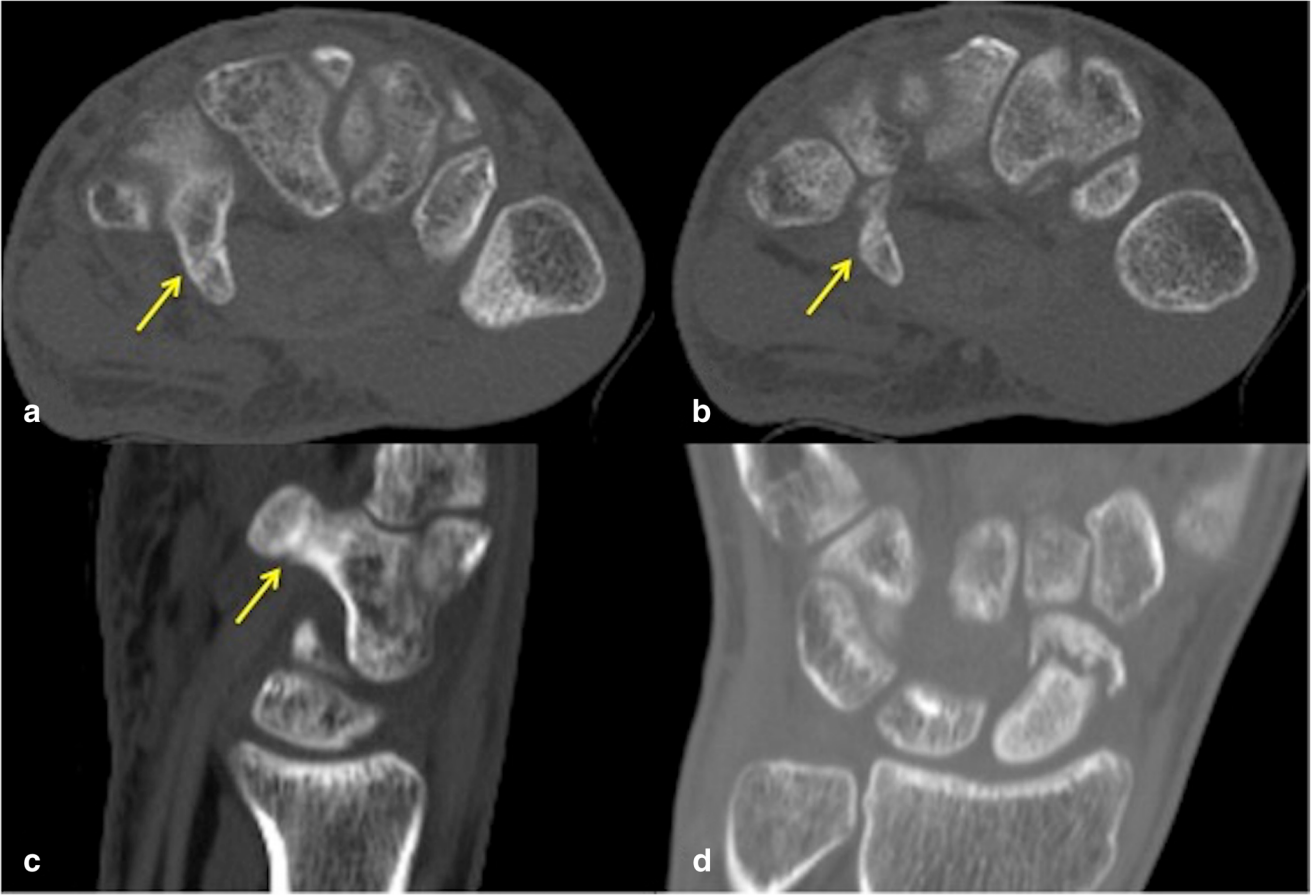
A 24-year-old man with fracture of the right scaphoid and concomitant hook of hamate fracture (CT performed 3 months after initial injury). a Axial CT bone window shows the subtle non-displaced fracture line across the hook of hamate waist (*arrow*), also seen in b at a slightly more distal level. Minimally and non-displaced hook of hamate fractures are often most conspicuous on axial images followed by c sagittal reformats. Note the marginal sclerosis in a–c suggestive of fracture healing. Interval CT 4 months later (not shown) confirmed a healed hook of hamate fracture. d Coronal reconstruction demonstrates the poorly healing distal pole scaphoid fracture with some sclerosis of the proximal component
